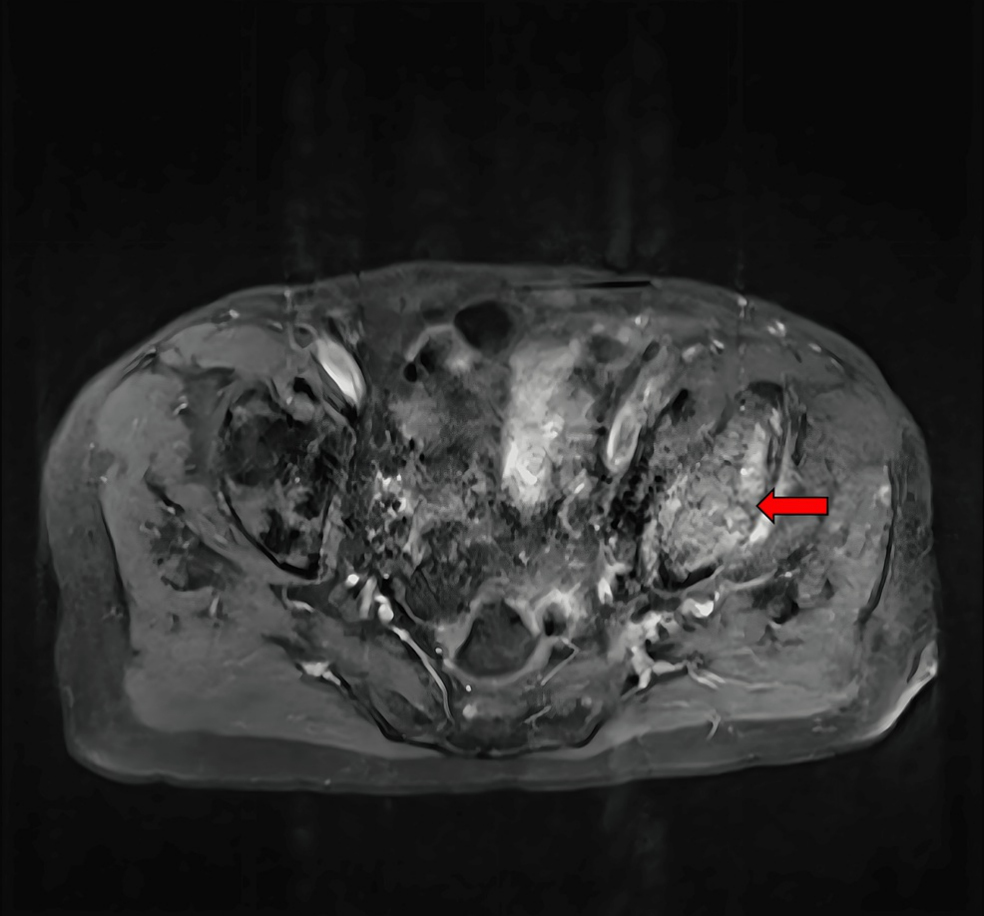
T1-weighted pelvic MRI with contrast, axial view An expansive enhancing lesion consistent in location, with the initial mass seen on a CT scan measuring greater than 6 cm in diameter, which is notably larger than originally being measurable on the CT scan, is present within the superoposterior acetabular roof of the left bony pelvis involving both the iliac and ischial segments of the hemipelvis (arrow). Cortical thinning is also present and indicates the potential for pathologic fracture with unassisted ambulation. The full image series reveals smaller enhancing lesions within the sacrum, iliac wings, and right femoral neck.
